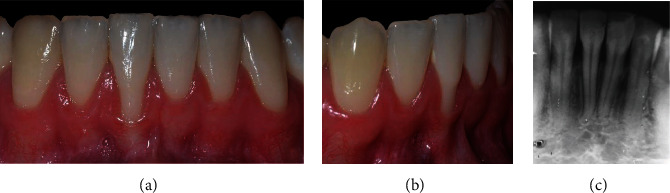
Case 1. (a) Frontal view, gingival recession of 4 mm high by 3 mm wide. (b) Right lateral view. (c) Periapical radiograph, 2 mm radiographic interproximal bone loss mesial and distal.
